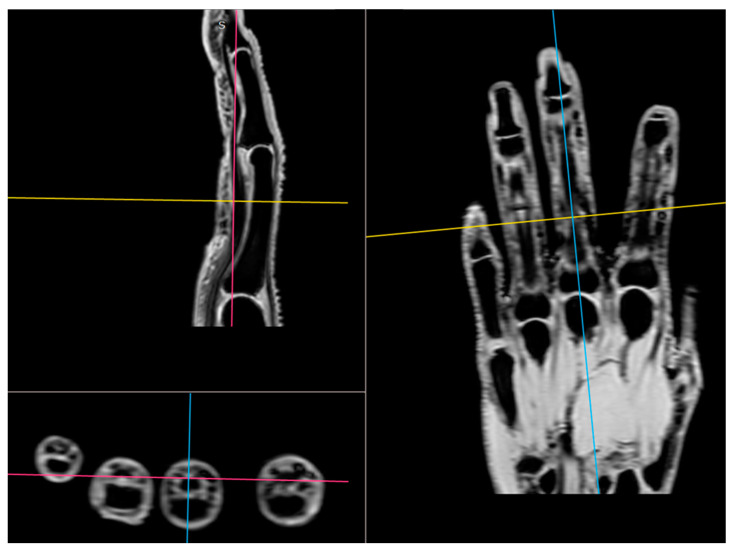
The application of a 3D sequence of MRI to obtain the true sagittal cross-section of each finger.
